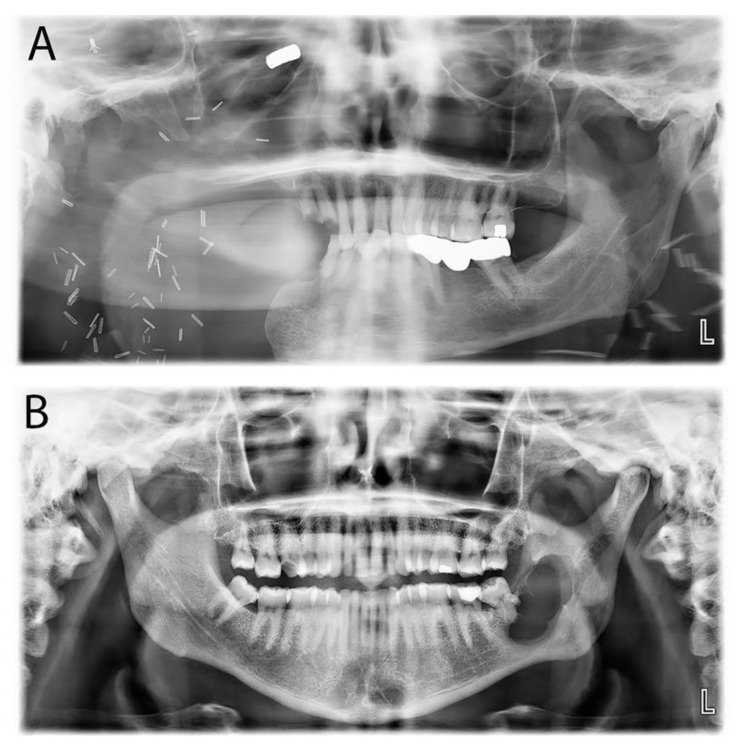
Examples of radiographic presentations of recurrent ameloblastoma. (A) Recurrent ameloblastoma on the right posterior mandible post-resection. (B) Recurrent ameloblastoma on the right posterior mandible post-excision.
